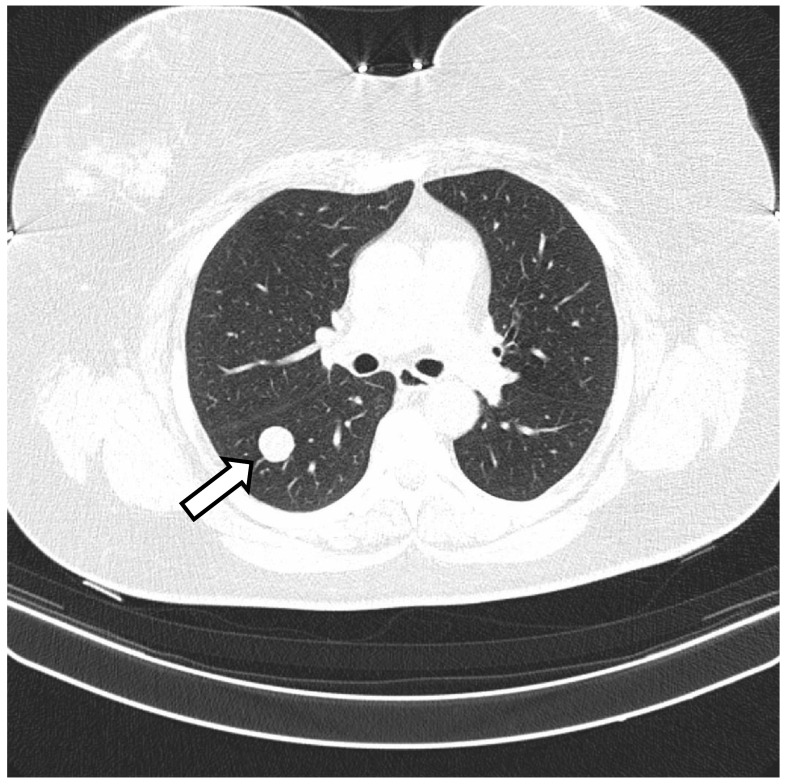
CT of the lungs. A single nodule is observed in the right lung (arrow).
